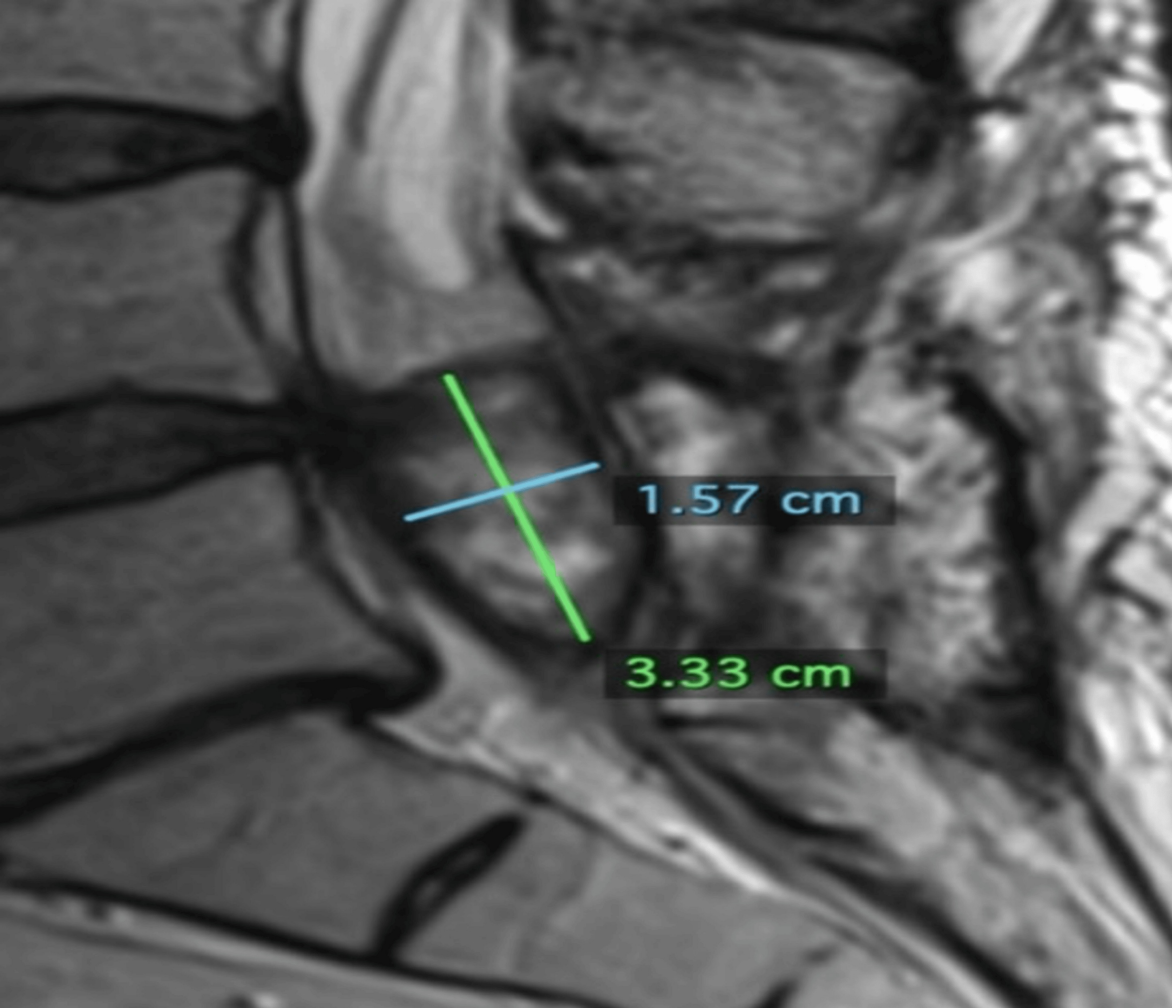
Sagittal image from an MRI L-spine with contrast obtained approximately two months post-cryoablation dated Aug 1, 2023. The tumor measures 3.33 cm superiorly to inferiorly and 1.57 cm anteriorly to posteriorly.
